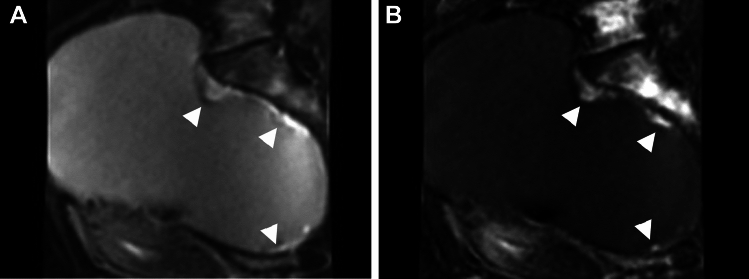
Endometriosis-associated ovarian carcinoma: clear cell carcinoma. A 47-year-old with suspicion of an ovarian cystic mass with mural nodules on ultrasonography examination. A On sagittal DWI (reduced field-of-view DWI, *b* = 800 s/mm^2^) a large left endometrioma with small mural nodules (arrowheads) is observed. The high signal intensity of the cyst contents masks the signal of small mural nodules and B computed DWI (*b* = 2000s/mm^2^) clarified the high signal intensities of the small mural nodules (arrowheads)
